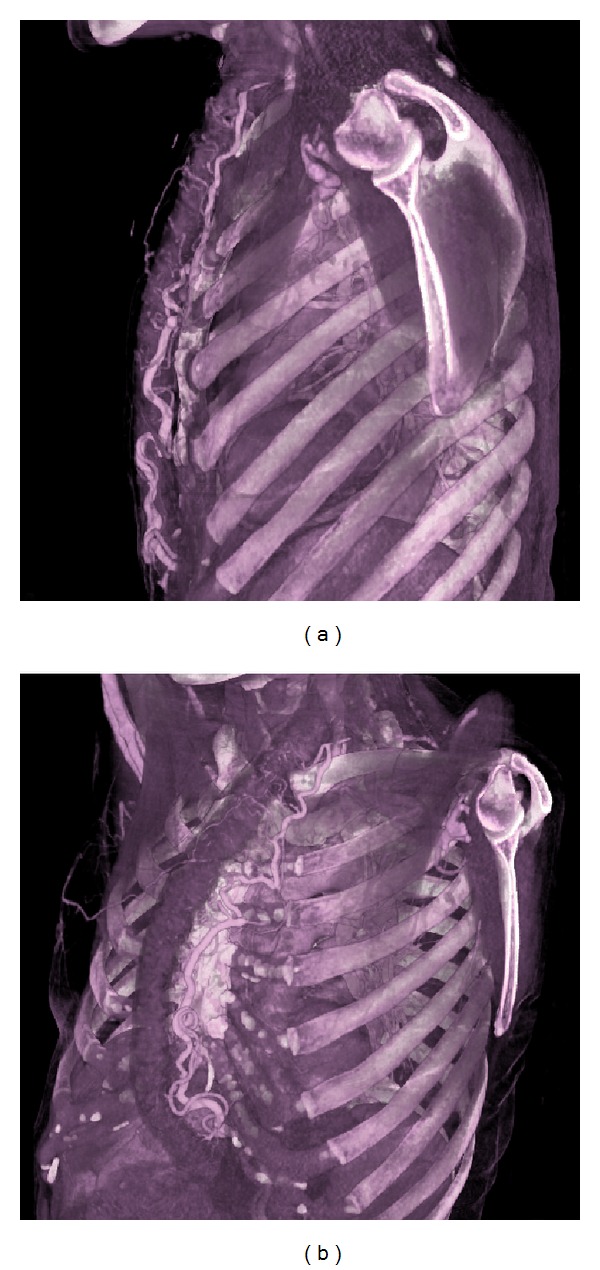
((a)-(b)) CT images with volume rendering reconstruction show the vascular anastomosis condition without stenosis or low-level perfusion signs.
